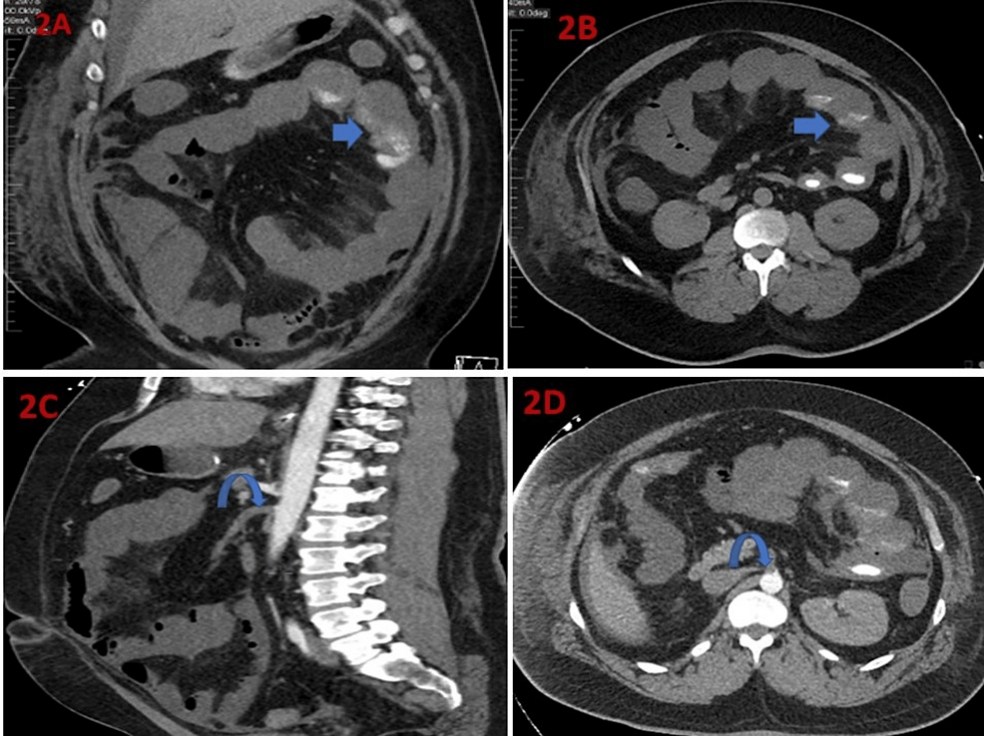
2A-2B: Non-contrast computed tomography scan of the abdomen; axial (A), and coronal (B) images of abnormal appearing mildly prominent and fluid-filled small bowel loops starting from the duodeno-jejunal junction to the fluid-filled colonic loops (arrow) with cutoff at the splenic flexure, mesenteric edema, and interloop ascites. 2C-2D: Computed tomography angiography of the abdomen; axial (C), and sagittal (D) images demonstrated: thrombus with complete occlusion of the superior mesenteric artery at its origin.
